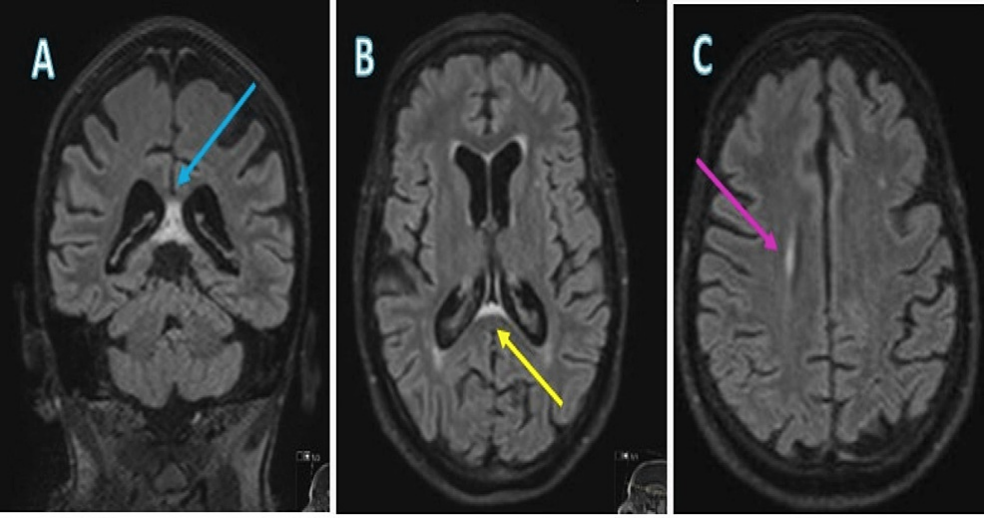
(A) Magnetic resonance imaging of the head coronal and axial fluid-attenuated inversion recovery images revealing hyperintensities within the corpus callosum (blue arrows). (B) Hyperintensities affecting the splenium and extending into the fornix (yellow arrows). (C) Non-specific white matter hyperintensities within the corona radiata (pink arrows).
